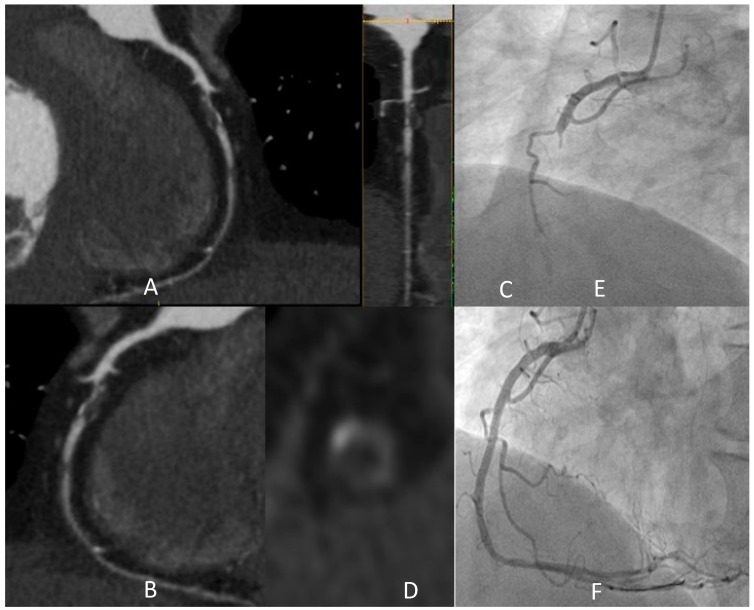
A 73-year-old male active smoker developed typical effort chest pain and CCTA was prescribed. Panel (A–D) shows a severe stenosis of medium RCA (after the origin of an acute marginal branch) characterized by prevalent lipidic plaque with all the typical HRP features: low attenuation (<30 HU in the central area), “napkin ring sign” (i.e., a low attenuating eccentric part surrounded by a thin, ring-like hyperattenuating rim), focal spotty calcification inside the lesion, and also positive remodeling. One other lipidic subocclusive stenosis is detected in the medium segment. In light of these findings, the patient was recovered to perform ICA in the following days. After an episode of chest pain at rest, urgent ICA was performed (E), showing a complete occlusion of the vessel in the segment involved by the abovementioned lesion, treated with PCI with multiple DES implantation (F). HRP: “high-risk plaque”; CCTA: coronary computed tomography angiography; DES: drug-eluting stent; HU: Hounsfield units; ICA: invasive coronary angiography; PCI: percutaneous coronary intervention.
